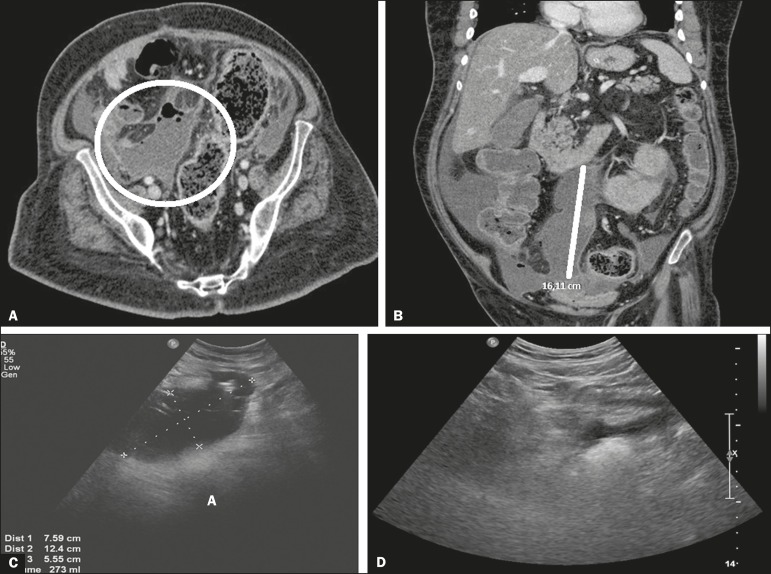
A,B: Computed tomography showing an intraperitoneal
